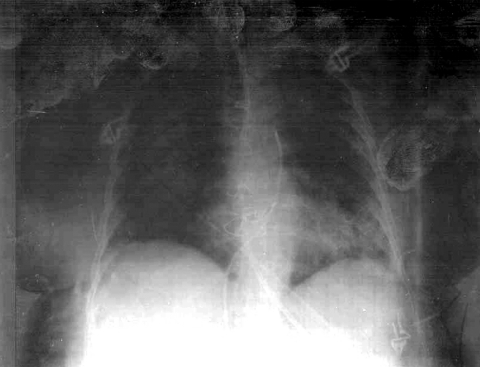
Chest radiograph of the patient showing bilateral alveolar infiltrates. Although pulmonary edema was the initial diagnosis, an infectious cause should be considered and, on the basis of sepsis, appropriate treatment initiated.
